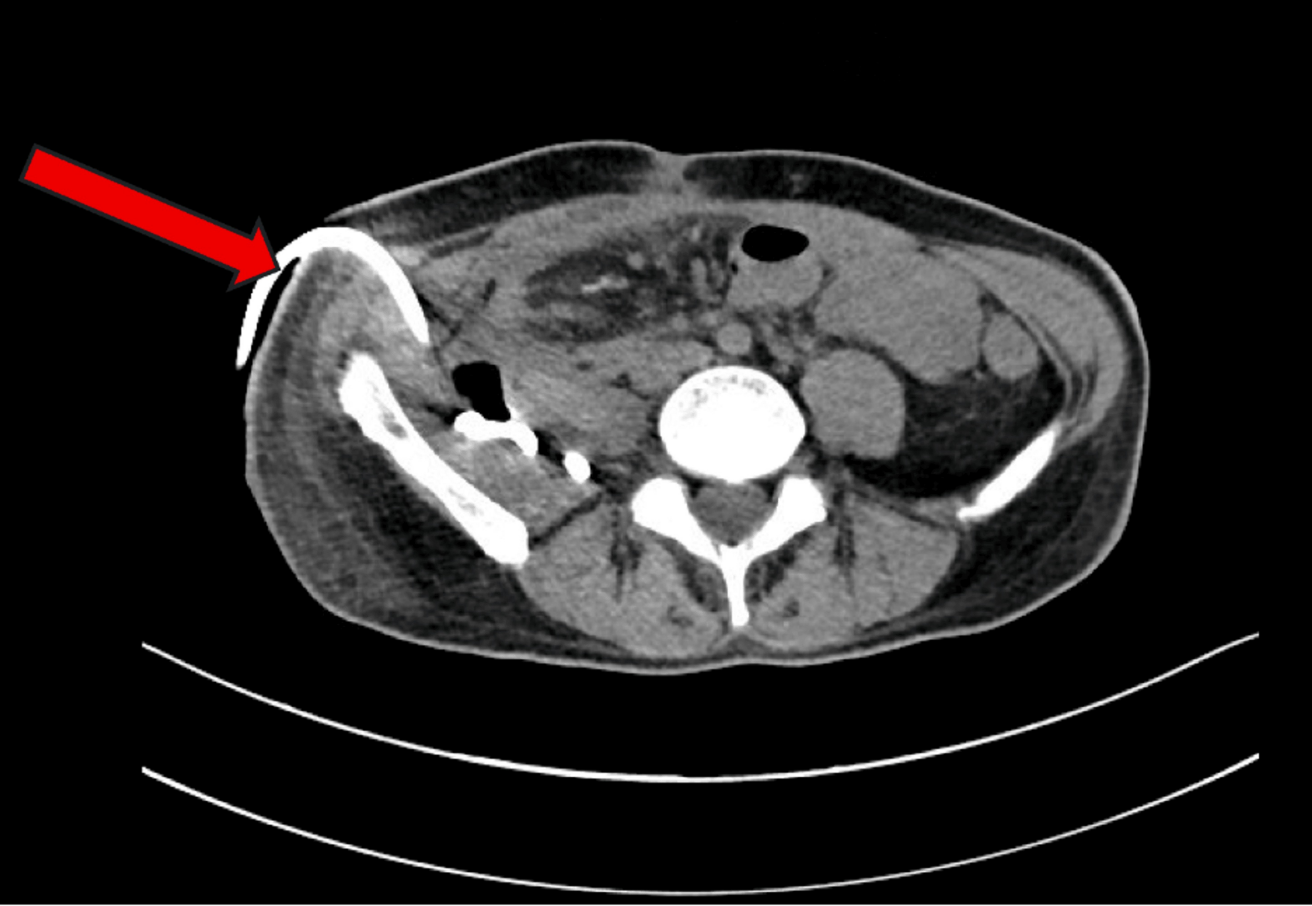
CT abdomen and pelvis without contrast axial view showing the presence of a right-sided retroperitoneal pigtail drain (red arrow), placed for a suspected collection.
